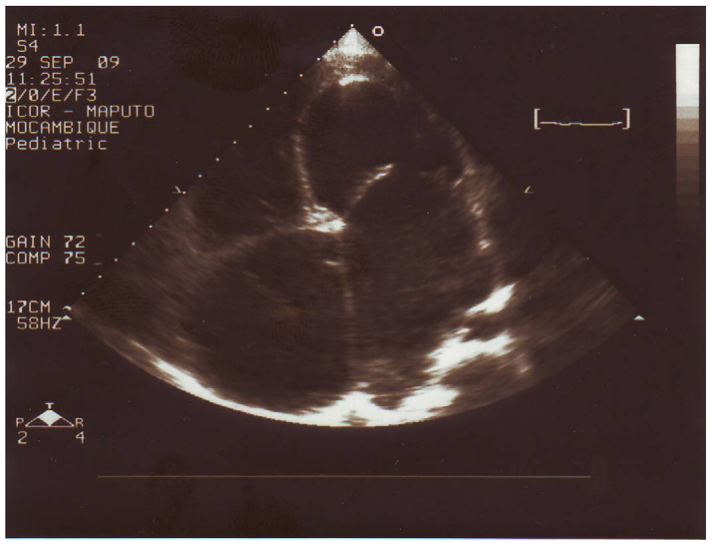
Severe left endomyocardial fibrosis. Echocardiography shows thickening of endocardium at the apex of the left ventricle that has a spherical shape and has reduced longitudinal dimension and thickening of the anterior leaflet of the mitral valve; left atrial dilatation is associated with both mitral regurgitation and reduced ventricular compliance. Notice the dilatation of the right cavities caused by elevation in pulmonary pressures.
